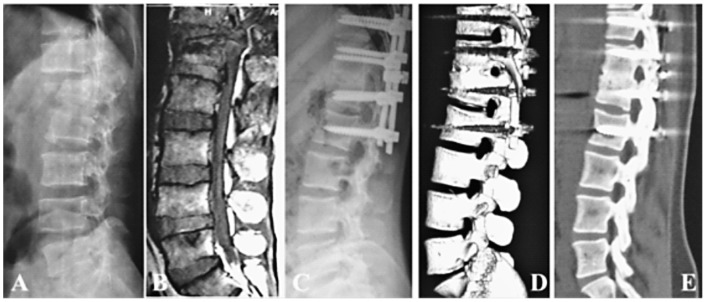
Type B1.2.2 fracture-dislocation in T12-L1 segment with disc rupture (A, B), posterior long-segment instrumentation, decompression, disc excision, and autologous local morselized bone graft in disc space in single-stage (C). Postoperatively 2 years, CT follow-up showing solid fusion in T12-L1 disc space (D, E).
